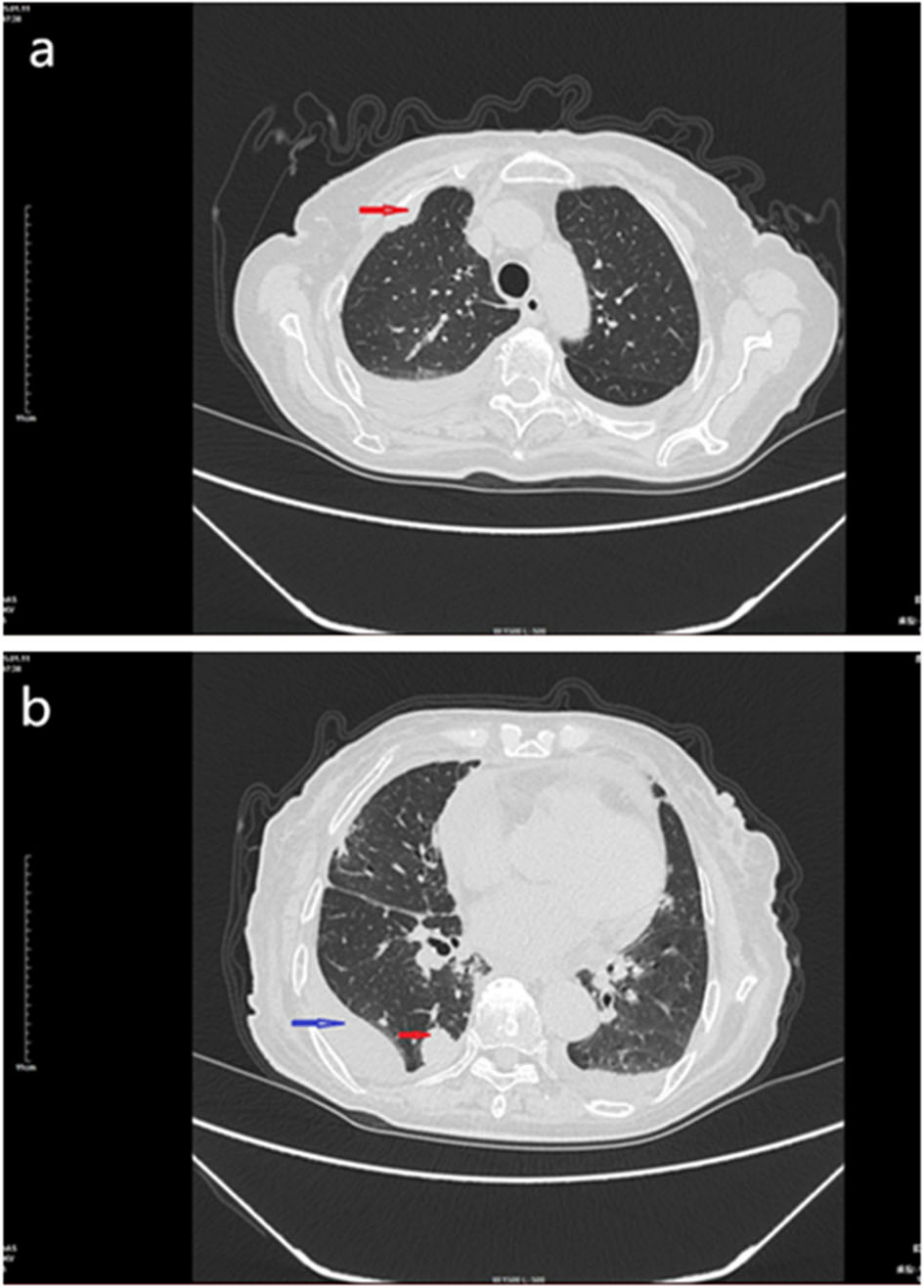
CT scan of the chest mass, January 11, 2015. (a) Enhanced chest CT showing a soft tissue mass in the right lung pleura (red arrow). (b) Enhanced chest CT showing a mass in the right lung (red arrow) and pleural effusion (blue arrow).
